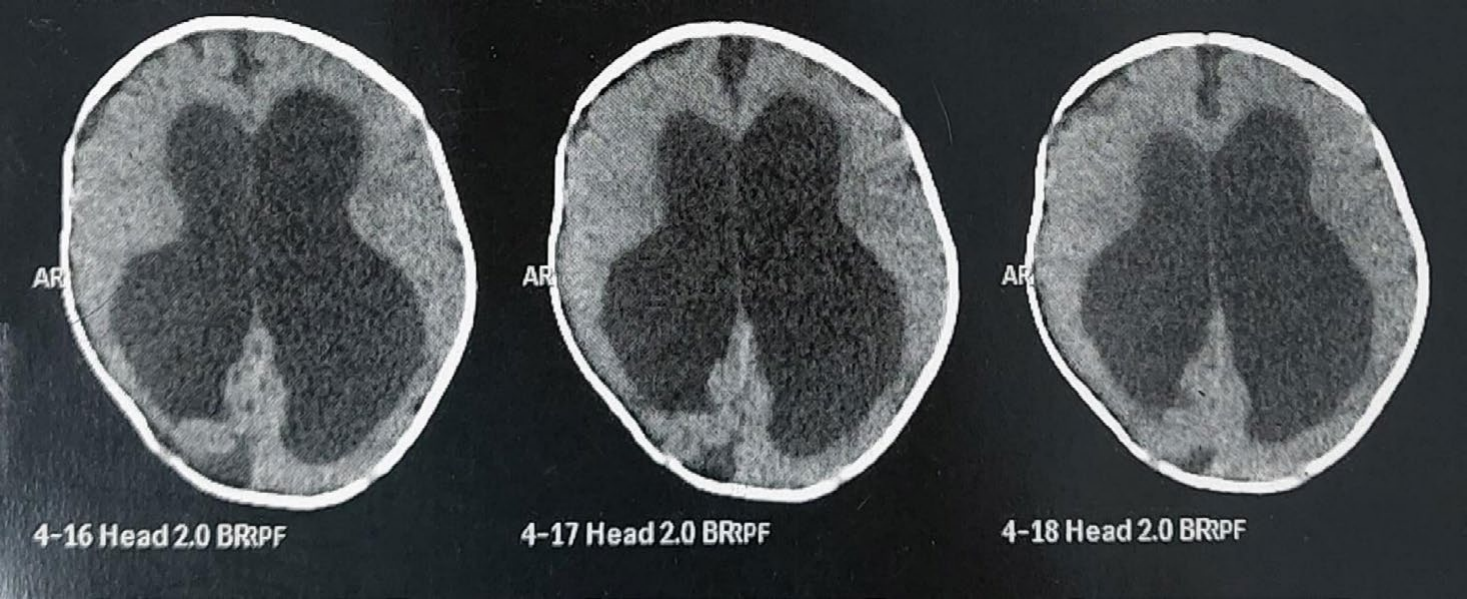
A CT scan showed the presence of extra‐axial hypodense areas on the right side of the brain, exerting a mass effect, compressing the ipsilateral ventricle, and causing a midline shift.
